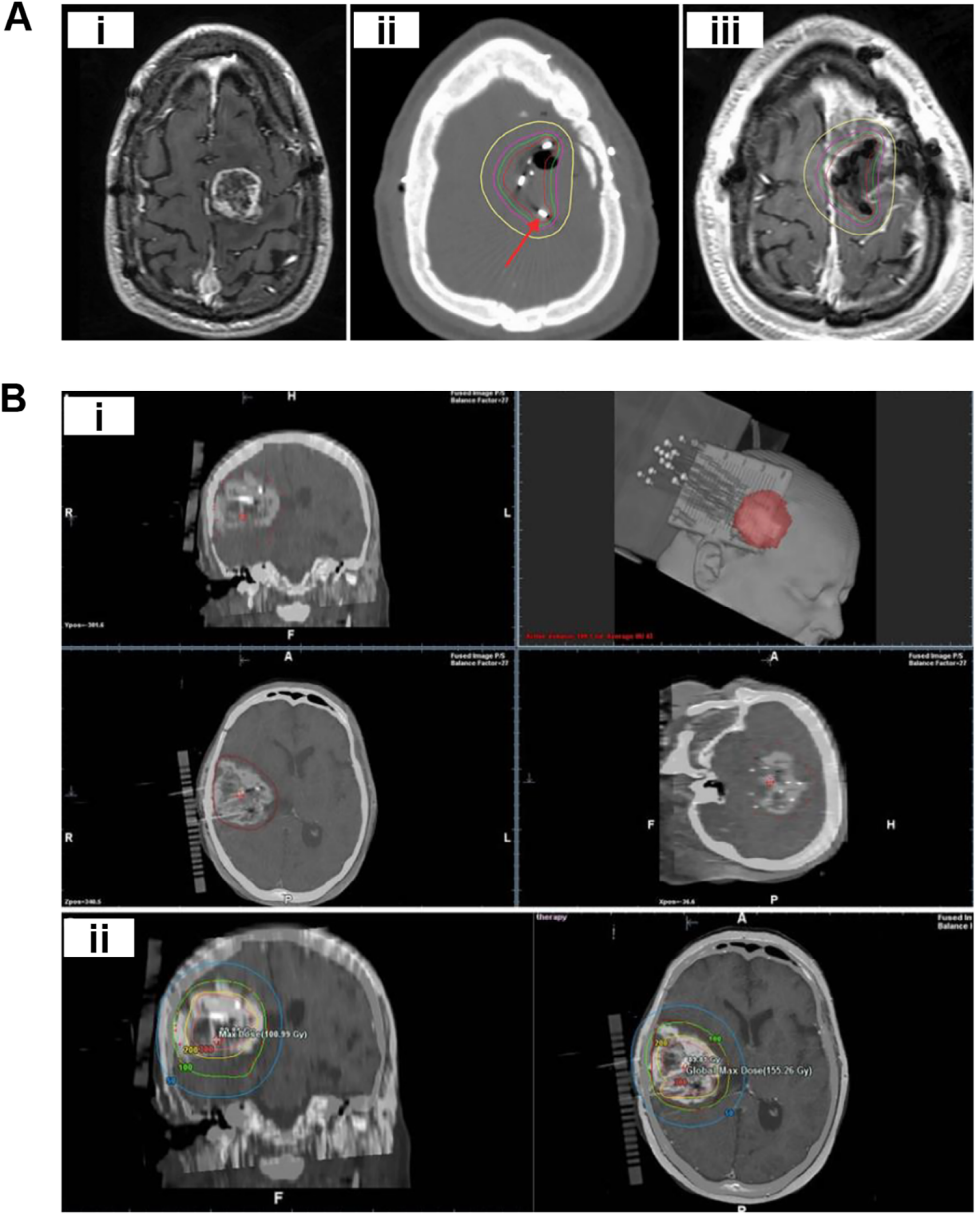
Brachytherapy for brain tumors. (A) Preoperative axial postcontrast T1‐weighted MR image (i). Postoperative axial CT image showing dosimetry with 30‐ (yellow), 60‐ (magenta), 80‐ (green), and 120‐Gy (red) isodose lines, and Cs‐131 seeds (arrow) (ii). Postoperative axial postcontrast T1‐weighted MR image with isodose lines as described in (B) and seeds appearing as small areas of signal void (iii). Reproduced under the terms of the CC‐BY 4.0 license.^[^
